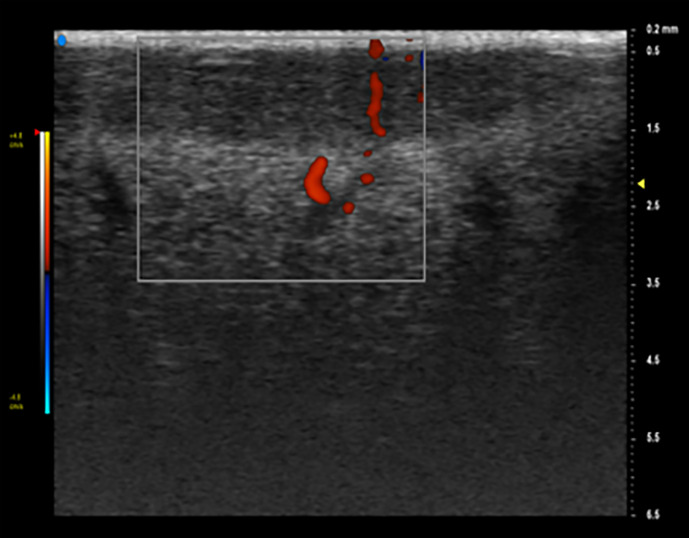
Doppler is an additional tool that can identify intra-tumor vessels and characterize their distribution, improving diagnostic accuracy. On Color Doppler examination, it is possible to see a hypoechoic lesion with an increased vascular signal.
